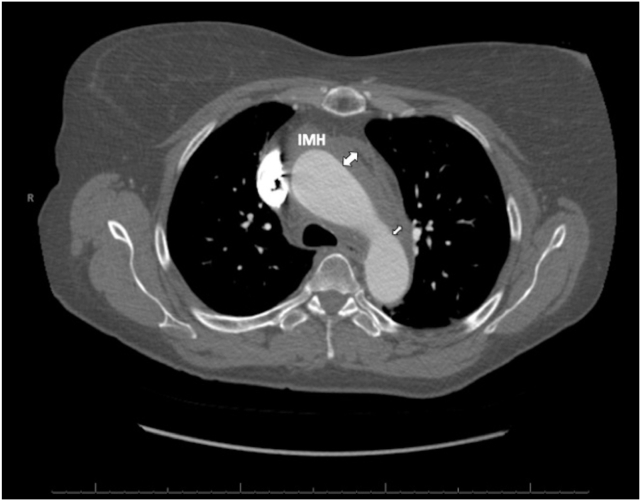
Contrast-enhanced CT scan, axial display at the aortic arch, demonstrates the superior extent of the IMH (*arrows*).
